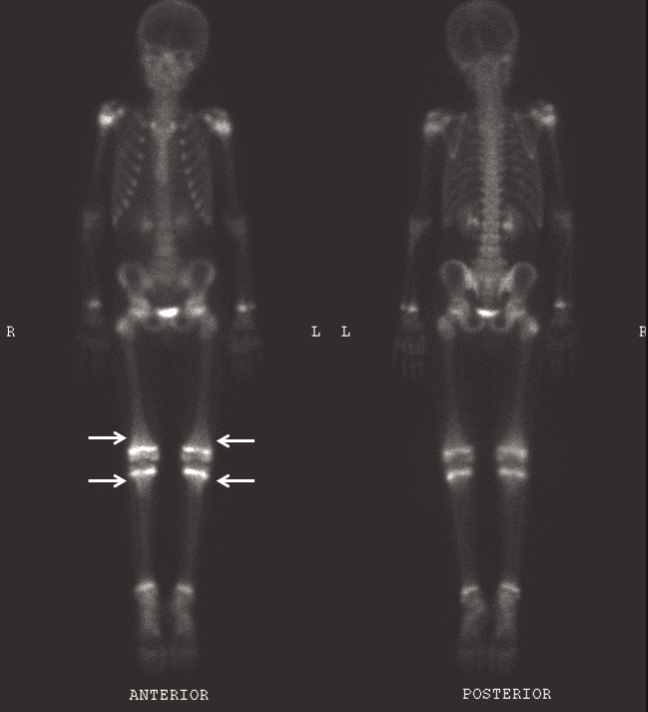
Whole-body bone scintigraphy demonstrates increased radiotracer activity in bilateral shoulders, wrists, hips, knees and ankles, most severe in the knees (*arrows*).
